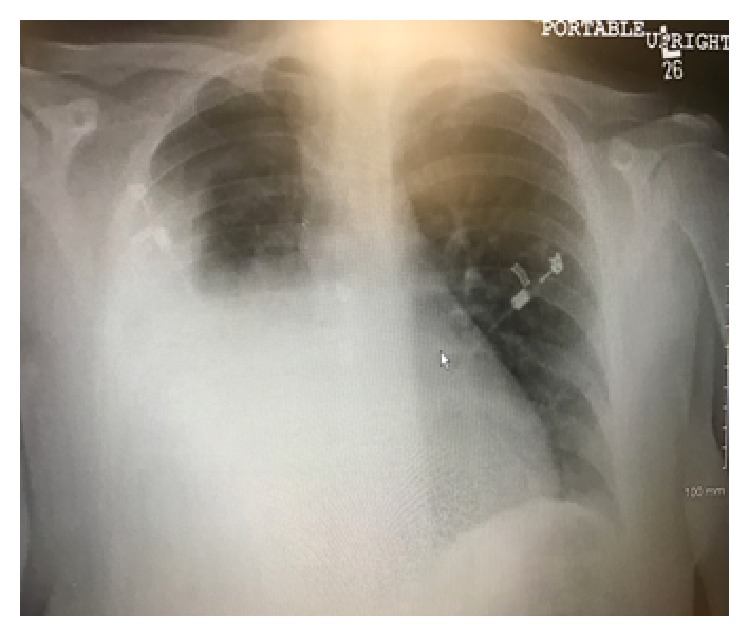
PA chest radiograph showing aeration of right upper lobe and postoperative opacification of the right lower lung field.
